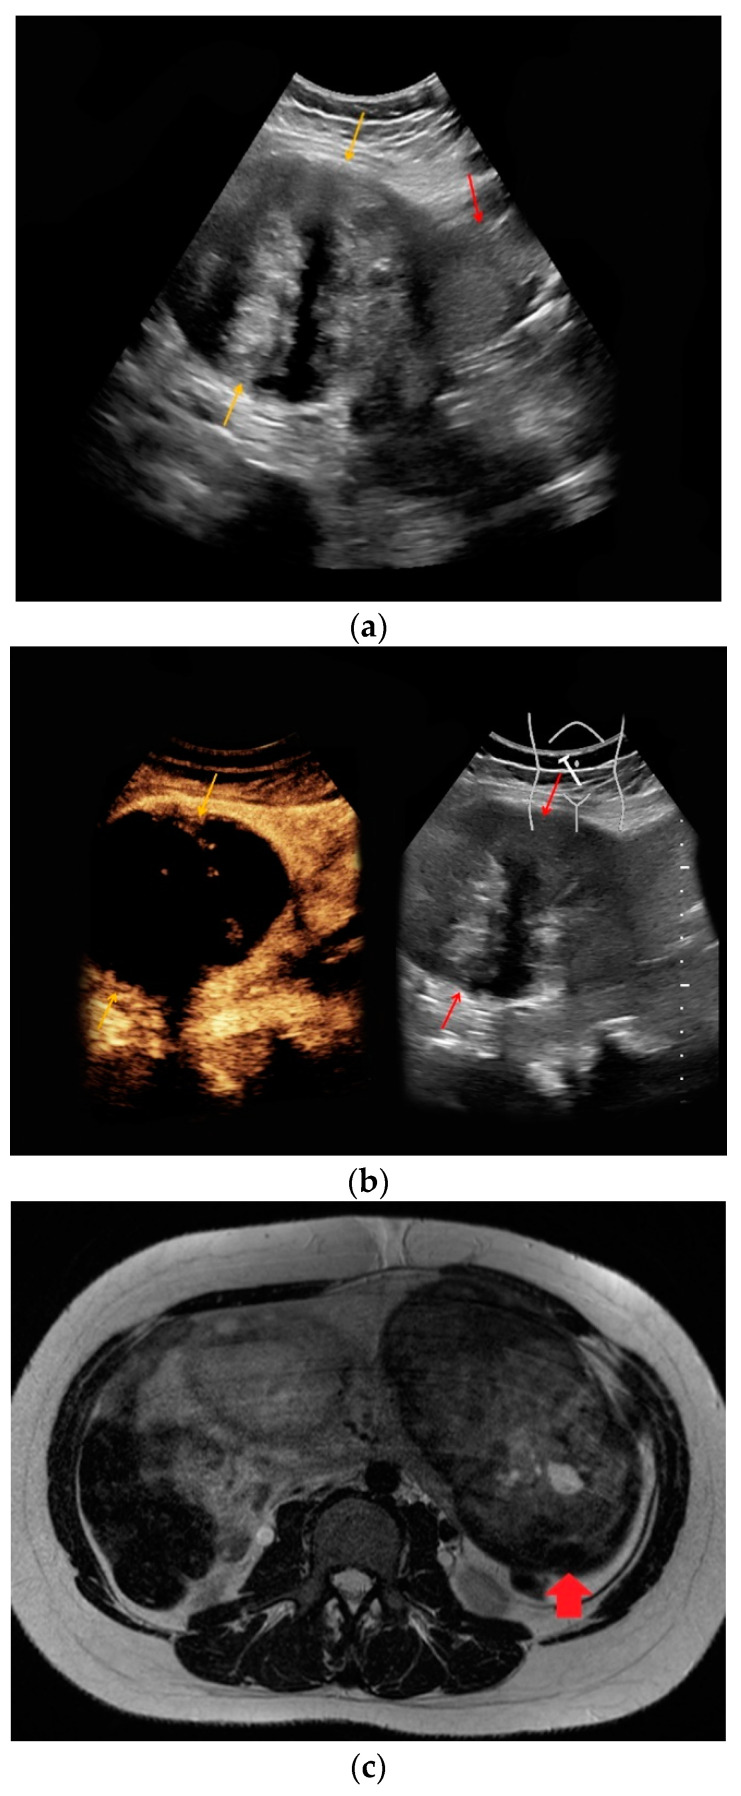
Necrotic uterine fibroid, 25 weeks of pregnancy. (a) Inhomogeneous, hypo-/hyperechoic lesion of the uterus with 15 cm in diameter in B-mode (yellow arrows; red arrow: uterus). (b) Only a slightly marginal contrast enhancement was detected in CEUS (yellow arrow). (c) In adjunct MRI, a predominantly T2-hypointense, inhomogeneous lesion of the left uterine wall was visualized (red arrowhead).
